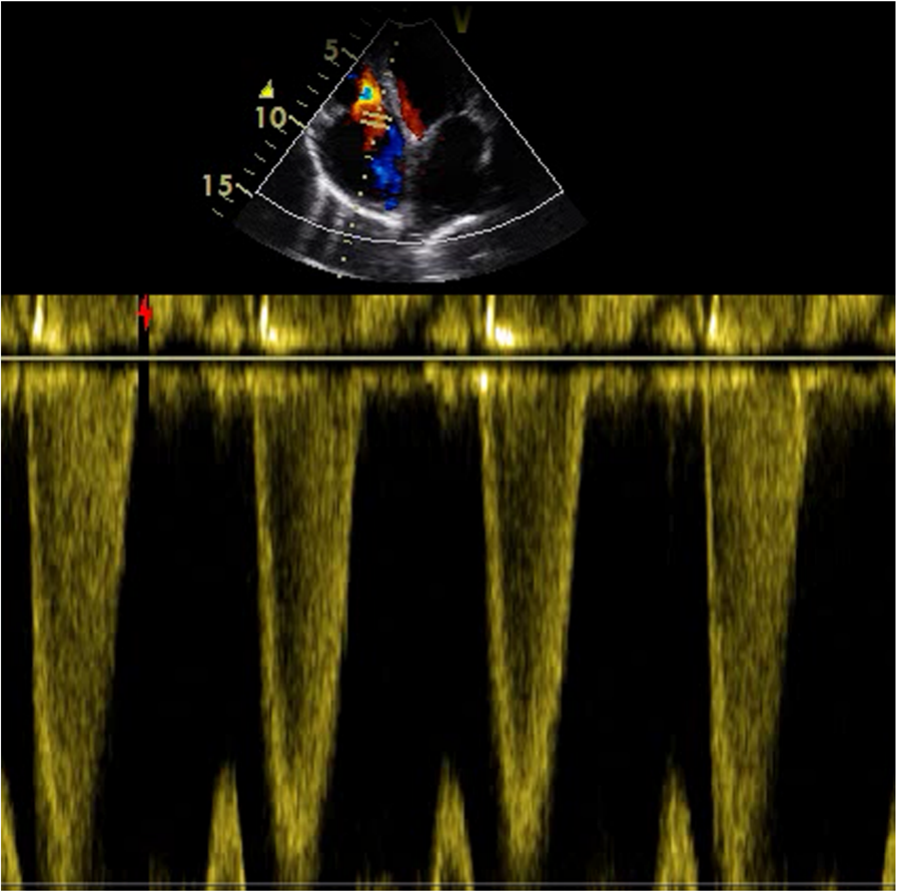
Doppler interrogation of tricuspid regurgitation showing laminar tricuspid insufficiency
